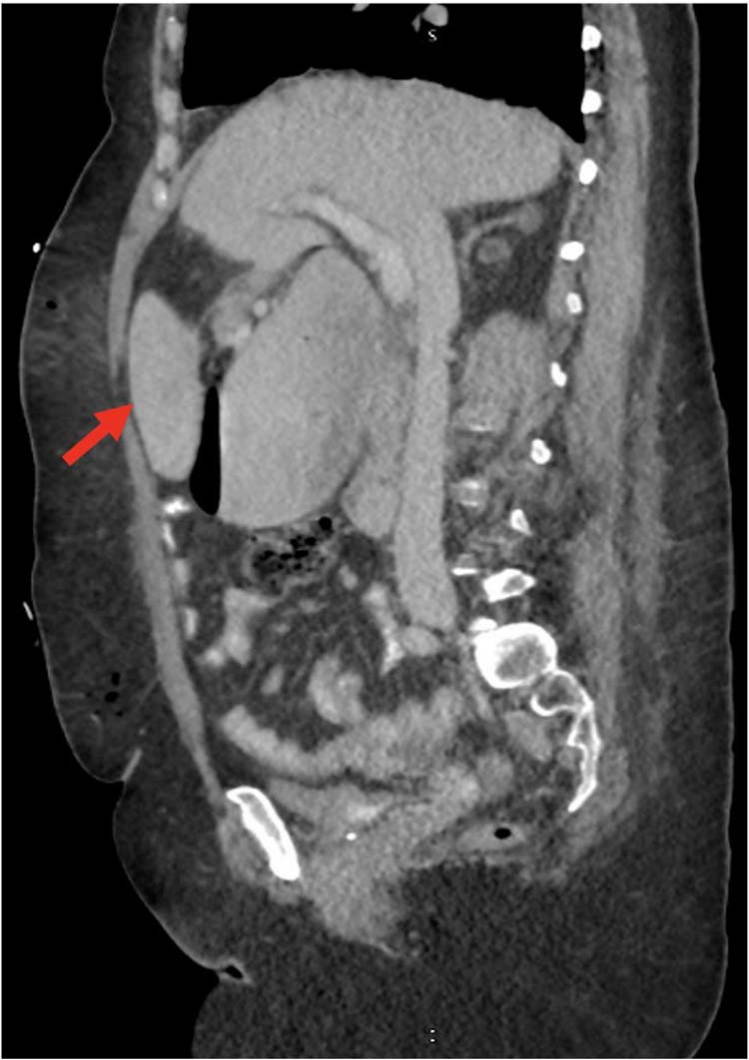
CT of the abdomen and pelvis (sagittal slice) showing gastric distention and the spleen positioned anteriorly.
